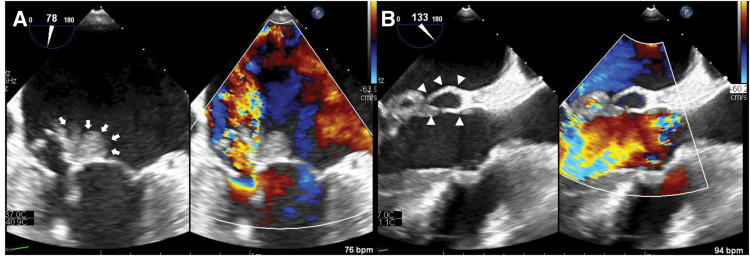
Preoperative TEE. TEE revealed an aneurysm of the anterior mitral leaflet with an intralesional perforation accompanied by a regurgitant jet (A, arrows). Severe AR and an echo-free space suggestive of an annular abscess beneath the NCC are also observed (B, arrowheads). AR, aortic regurgitation; NCC, non-coronary cusp; TEE, transesophageal echocardiography
